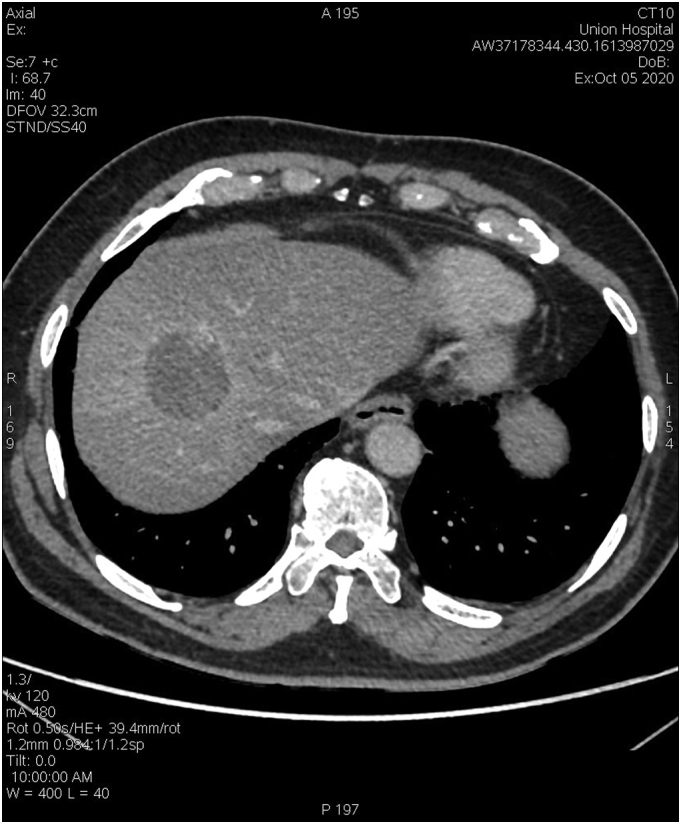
pre-operative CT scan, no visible middle hepatic vein was shown.
